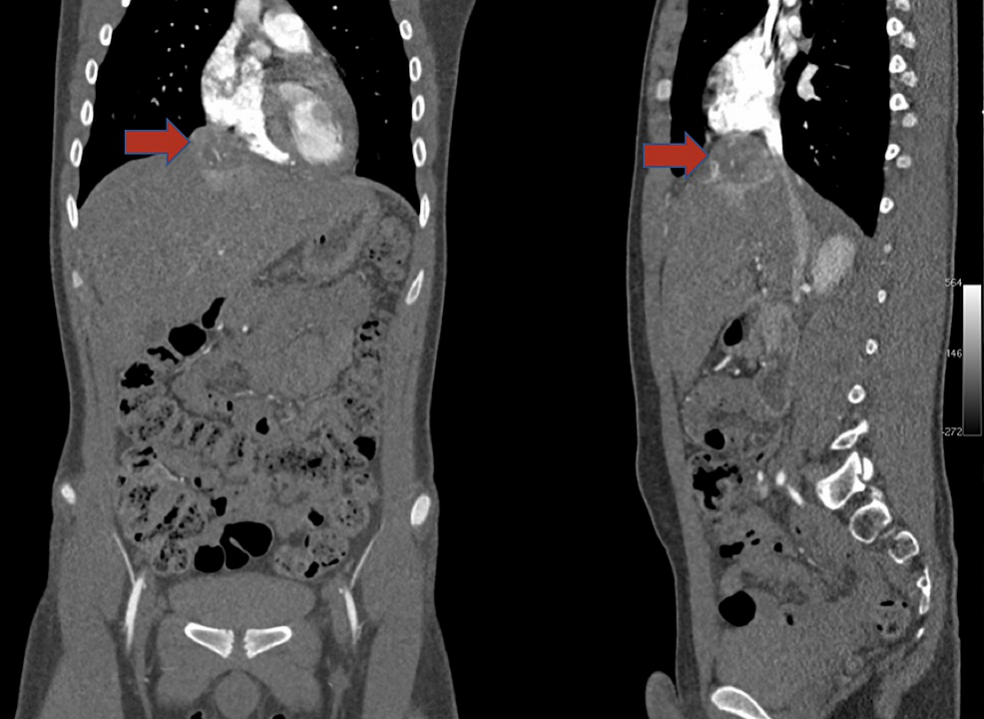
Abdominal computed tomography revealed a solid lesion located between the liver capsule and the diaphragm. The lesion appeared to be in close relation with the cavoatrial junction.
